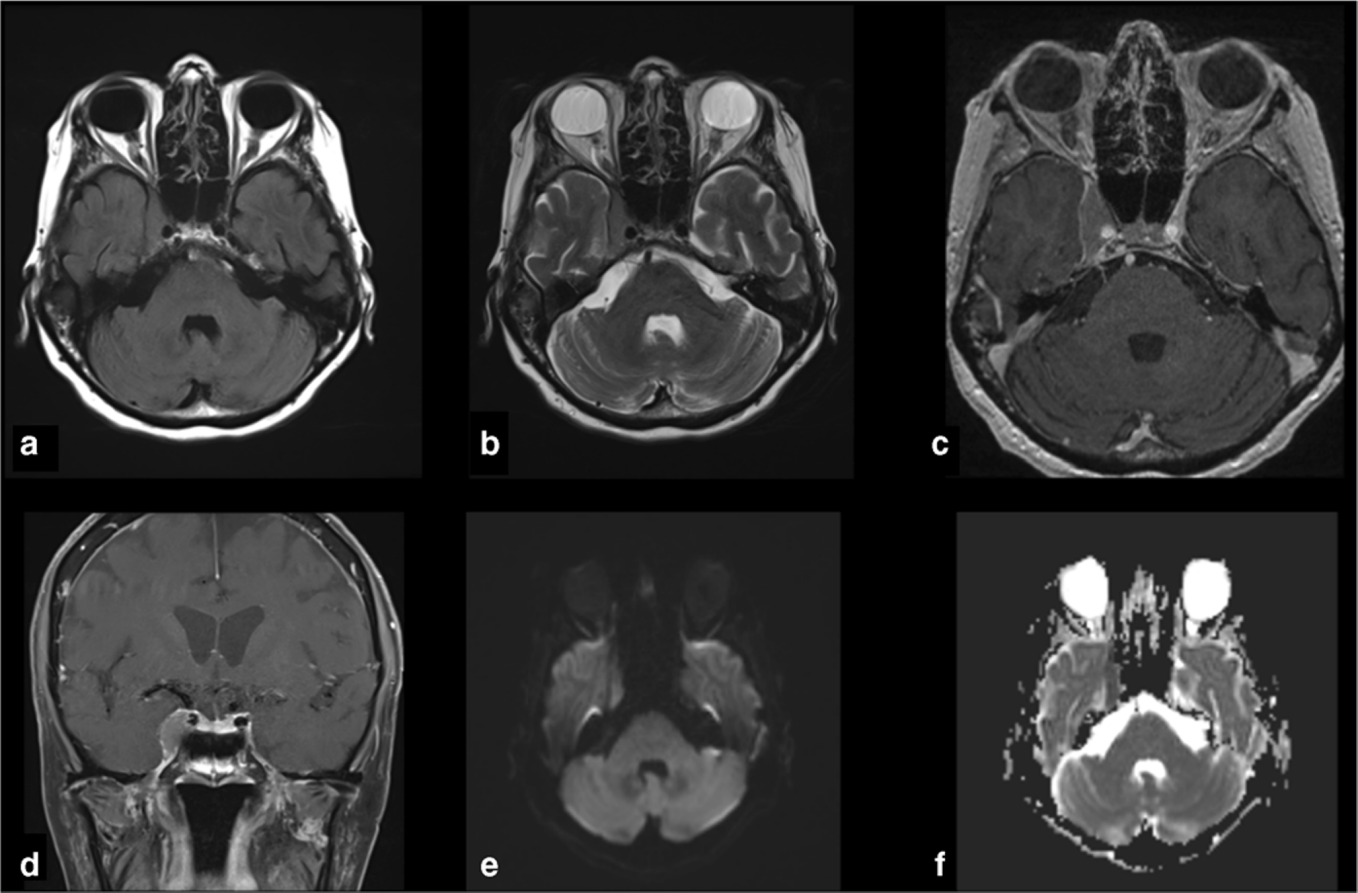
Preoperative imaging of a 78-year-old female patient harboring a lesion in Meckel’s cave. No hyperintensity was observed in the a FLAIR- or b T2-weighted scan. There was however contrast-enhancement demonstrated in the c axial and d coronal T1-weighted imaging. No diffusion restriction could be seen in the e DWI, but hypercellularity was demonstrated in the f ADC-sequence
